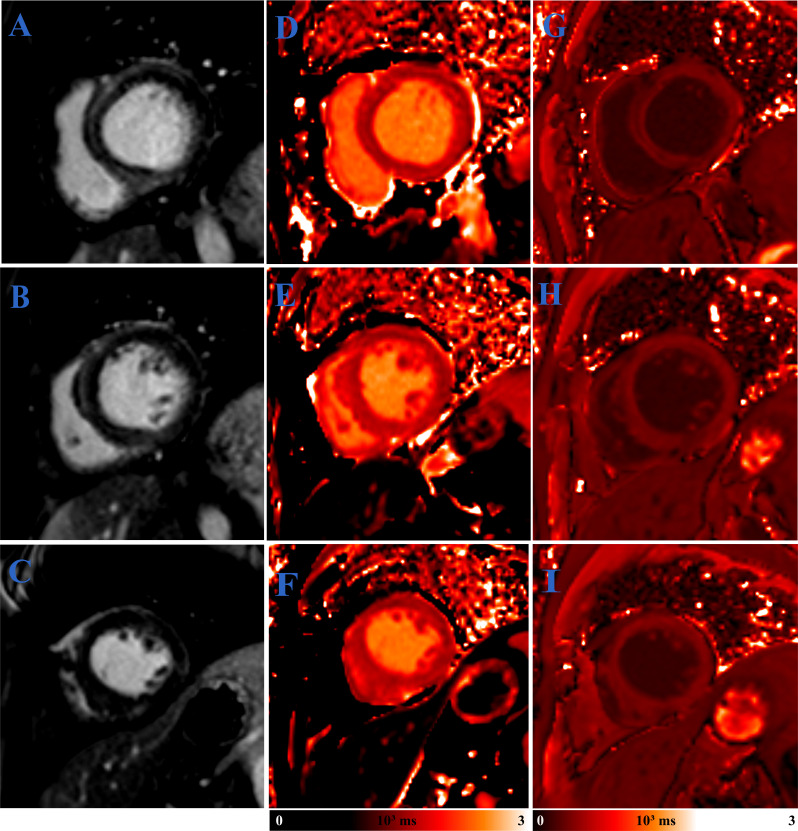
Typical cardiovascular magnetic resonance images from a
